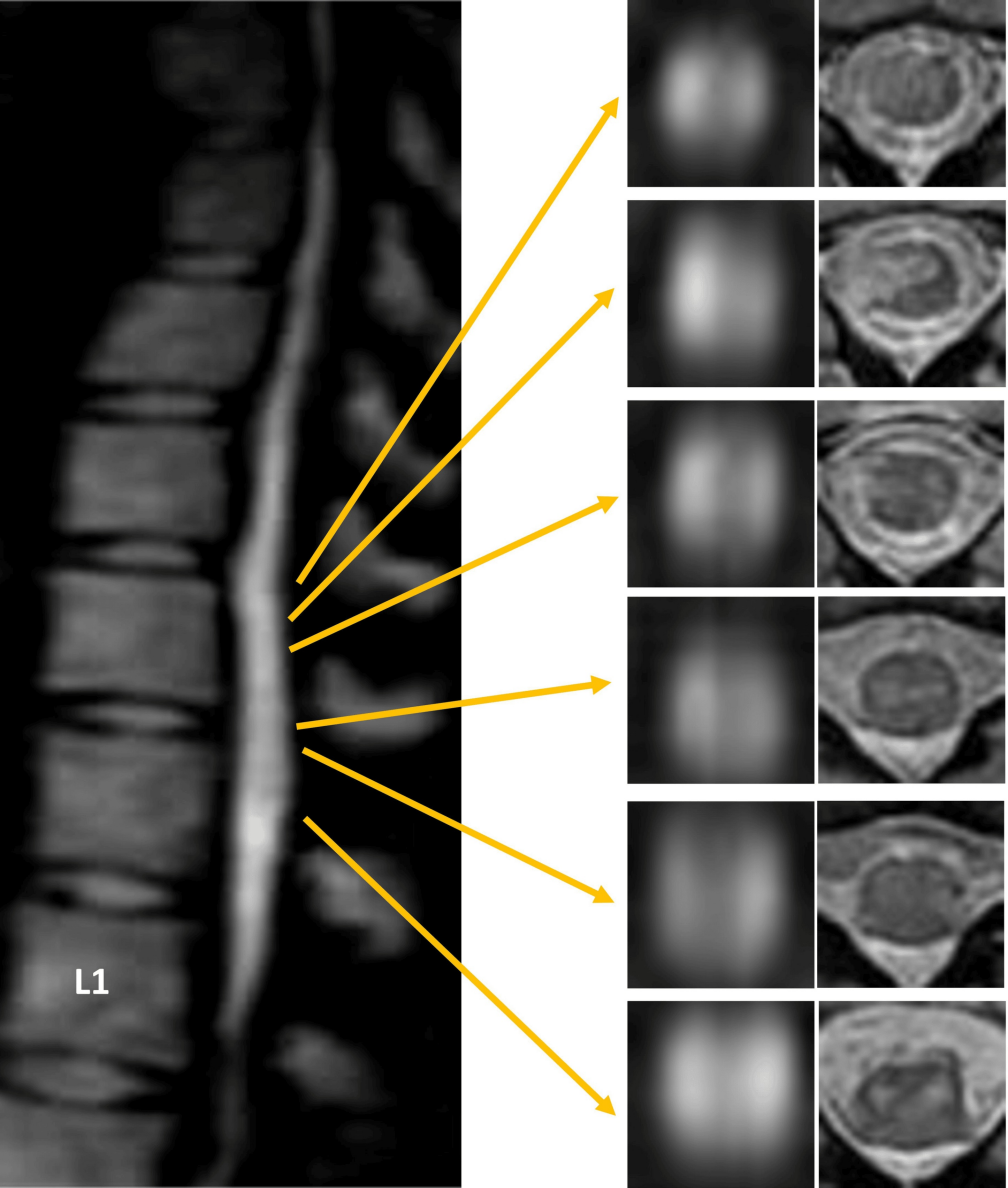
The result of thoracic MRI on diffusion-weighted and T2-weighted imaging on day three. Diffusion-weighted and T2-weighted thoracic MRI show hyperintensity in the spinal cord at the Th10 to Th12 levels. Axial sections of diffusion-weighted imaging reveal the "owl sign" between the Th10 and Th12 levels. The sagittal section is shown using diffusion-weighted imaging. The axial section to the left also shows diffusion-weighted imaging. The axial section on the right demonstrates T2-weighted imaging.
